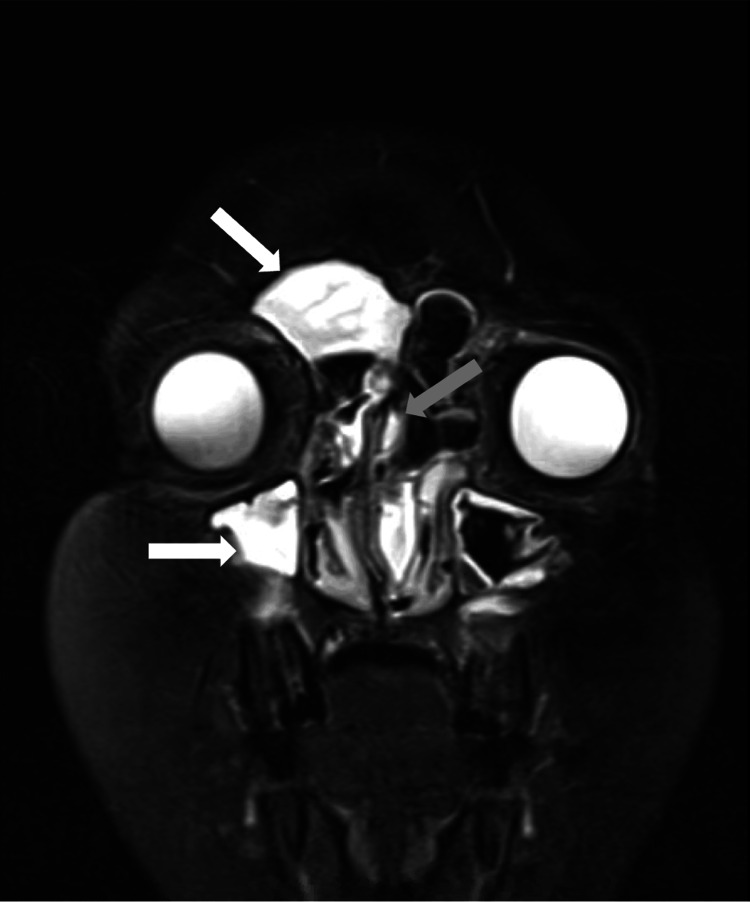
Coronal T2-weighted MRI of face and neck The image demonstrates diffuse hyperintense signals within the paranasal sinuses. There is opacification of the right frontal sinus and right maxillary sinus (white arrows). Expansion of the right and left ethmoid sinuses is demonstrated as well (grey arrow) MRI: magnetic resonance imaging
